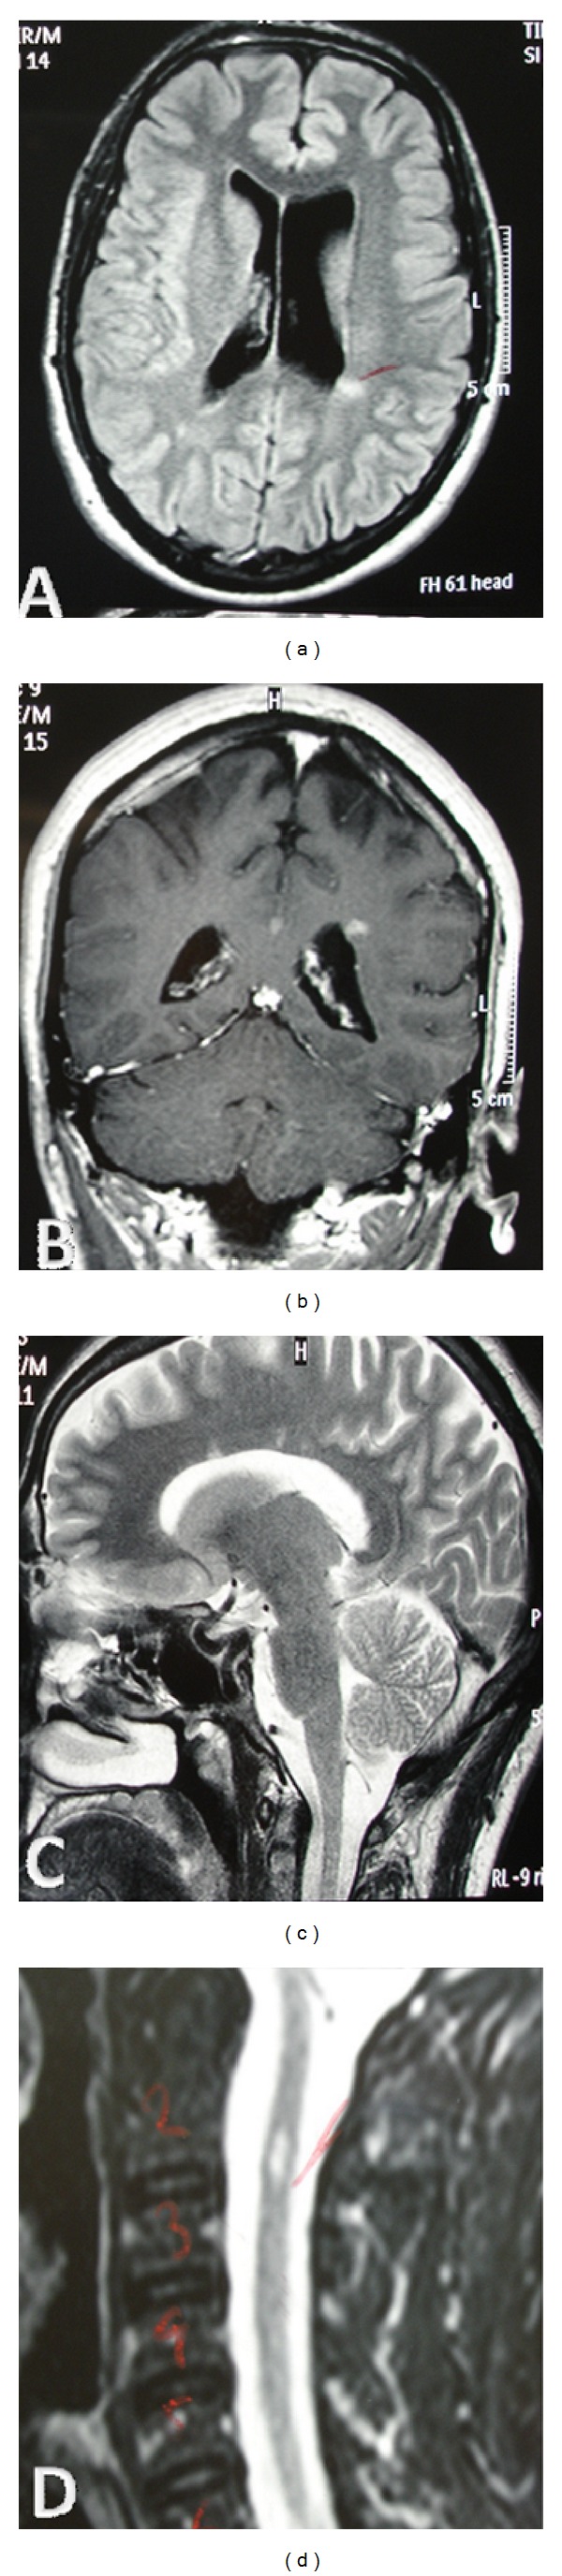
Magnetic resonance imaging of the brain and spinal cord at first attack, showing lesions indicative of multiple sclerosis. (a) Axial FLAIR image showing hyperintense lesions in the white matter of both hemispheres. (b) Coronal T1-weighted image showing a gadolinium-enhancing lesion near the left lateral ventricle. (c) Sagittal T2-weighted image showing lesions in the corpus callosum. (d) Sagittal T2-weighted image showing a hyperintense lesion on cervical spinal cord at C2 level.
